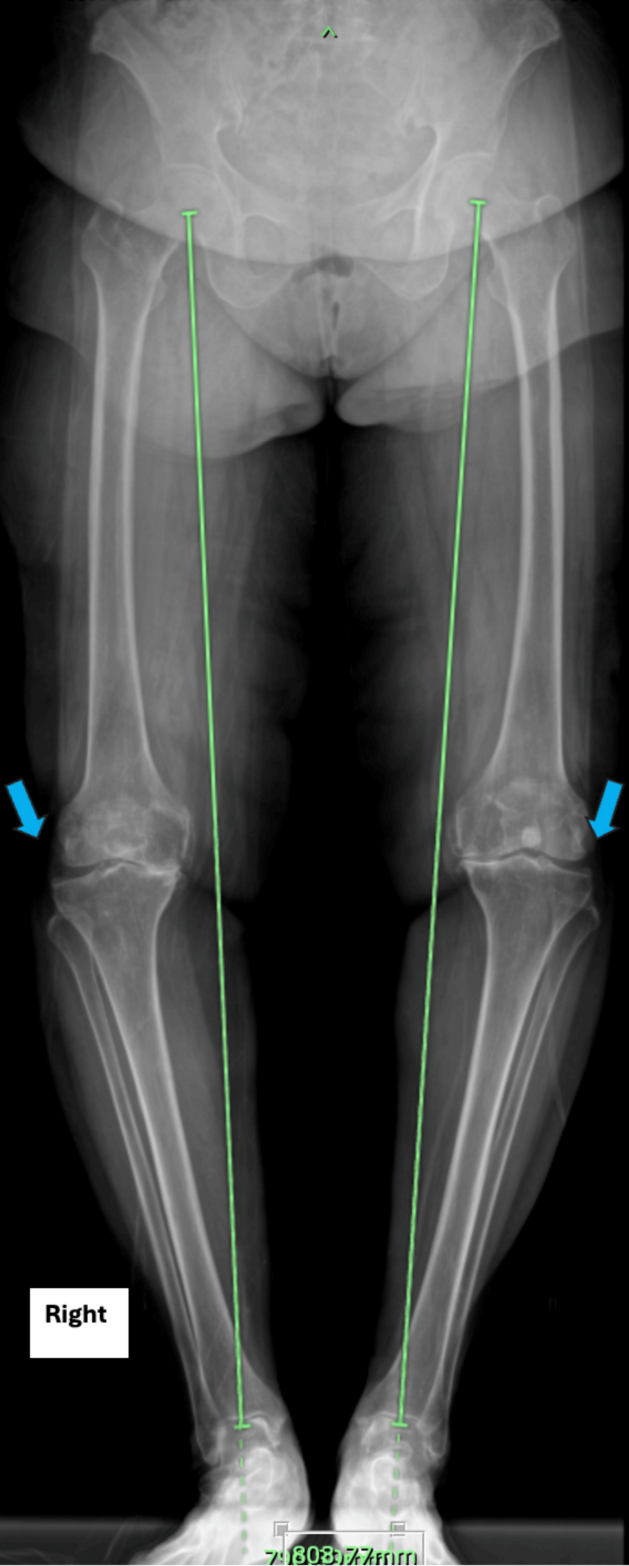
Plain X-ray of the bilateral lower limb showed genu varus deformity with osteoarthritis changes in the bilateral knee.
